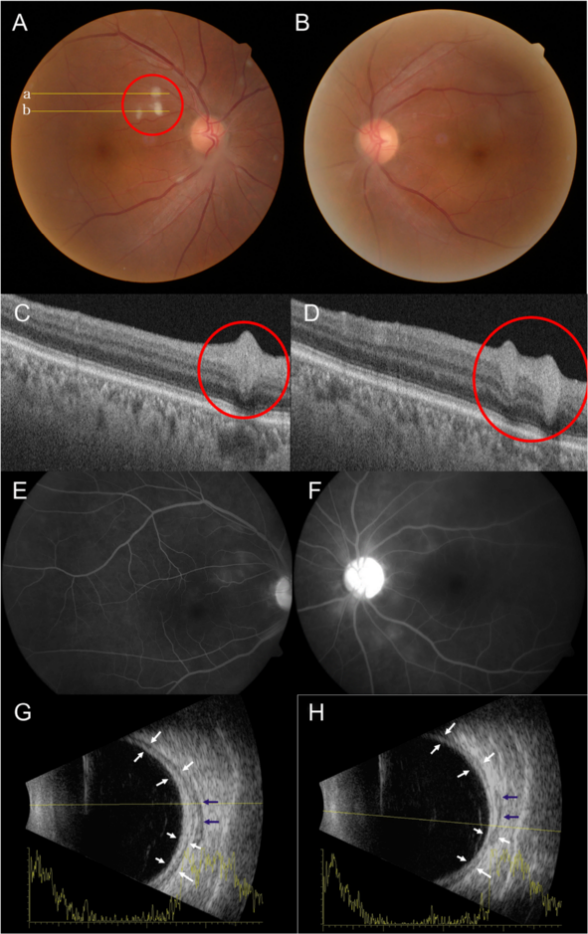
Suspect retinal granulomas (*red circles*) of the right eye at presentation. a Fundus photography showed three whitish plaques (*red circle*) on the right eye, mimicking cotton wool spots. b There was no lesion in the left eye. c Optical coherent tomography (OCT) scan, corresponding to line ‘a’, shows a well demarcated oval shape lesions without shadowing in retinal nerve fiber swelling (*red circle*). d OCT scan corresponding to line ‘b’ demonstrates two lesions (*red circle*). e and f Fluorescein angiography showing mild leakage around the optic disc in the late phase. Ultrasonography of the right eye (g) and left eye (h). White arrows indicate thickened sclera and black arrows point to fluid collection
